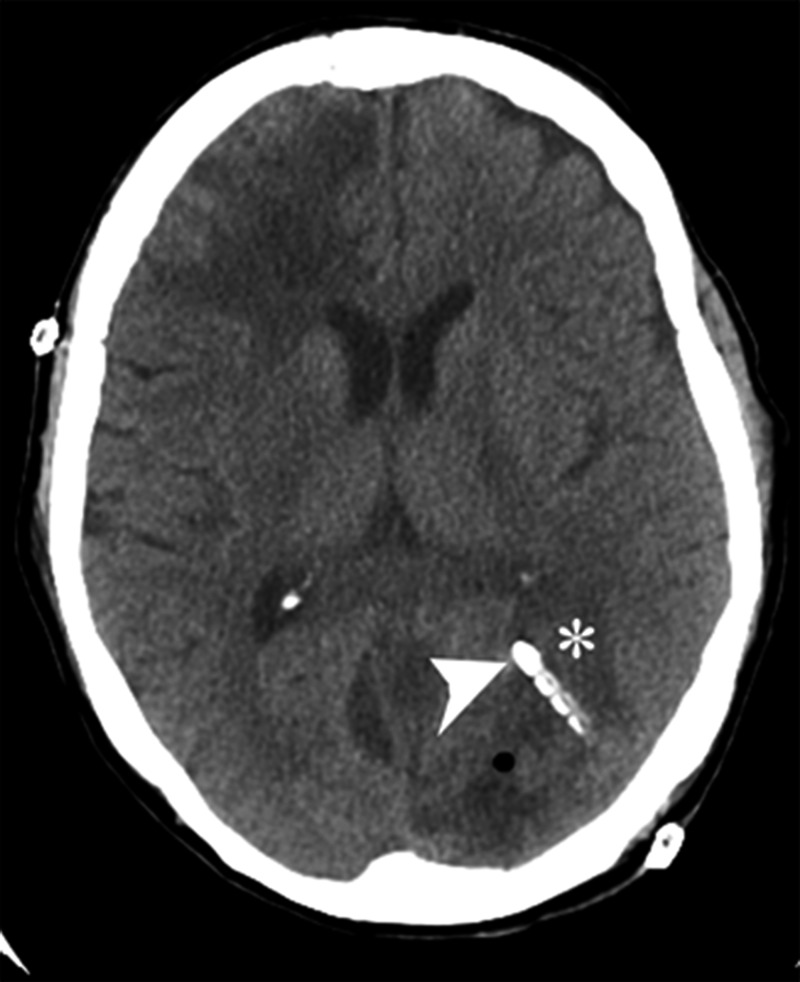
CT brain without contrast showing abscess and catheter. CT of the brain without contrast is shown with the abscess (*) penetrated by the catheter (arrow), through which vancomycin was injected for direct intra-abscess antibiotic treatment. CT: computed tomography
